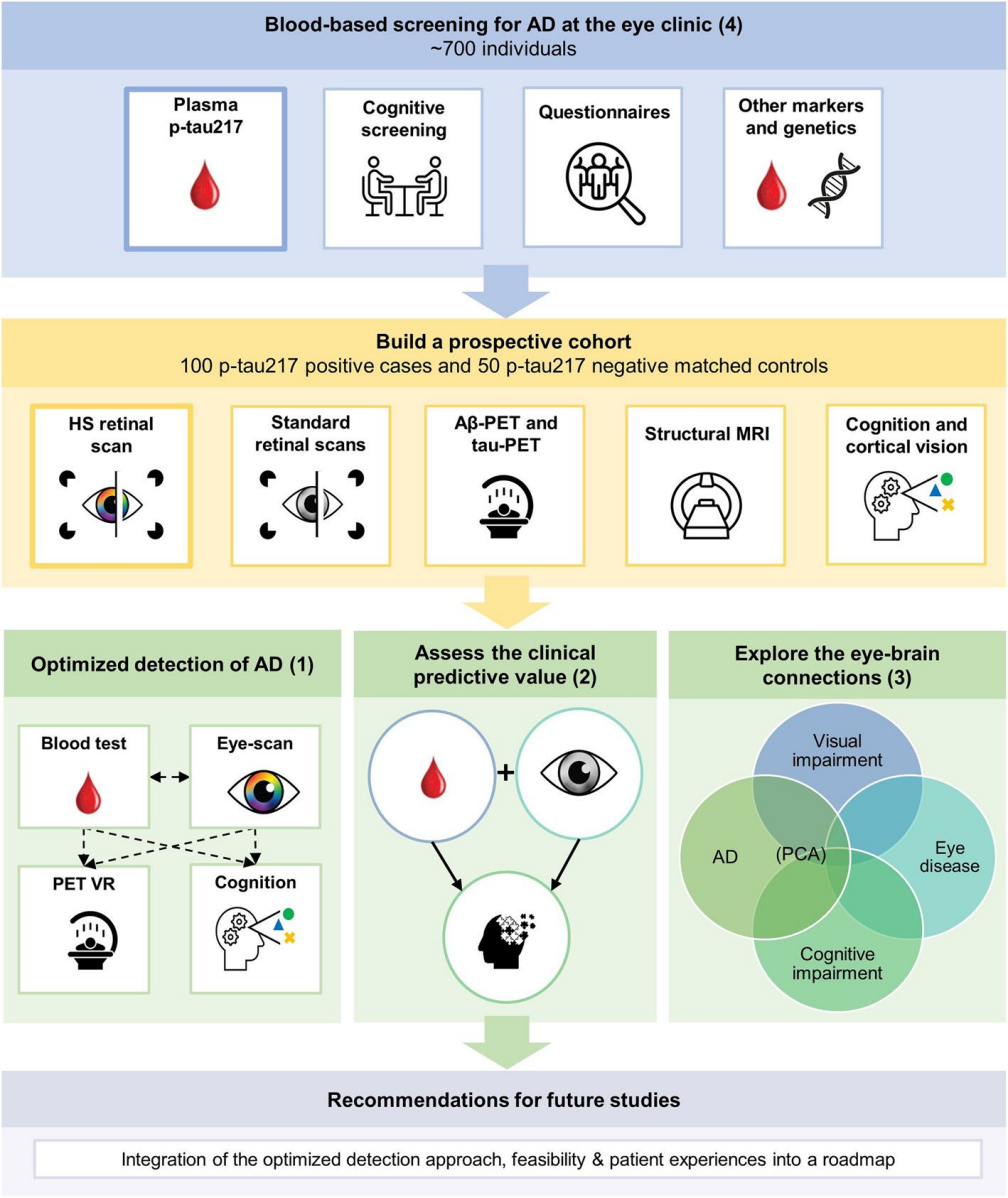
BeyeOMARKER study aims. *Abbreviations*: AD = Alzheimer’s Disease, PET = Position Emission Tomography, MRI = magnetic resonance imaging, Aβ = Amyloid beta, PCA = Posterior Cortical Atrophy
